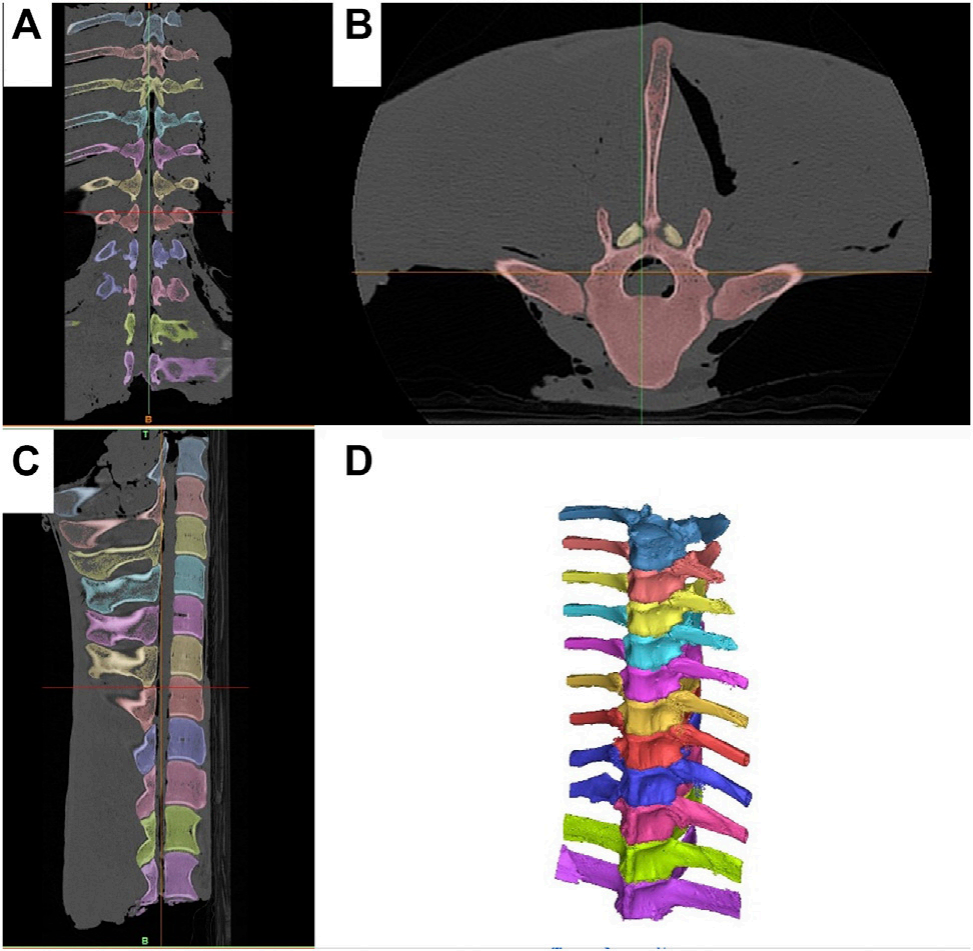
Segmentation of the T11-L1 spine segments. Each vertebra is segmented separately and denoted in a different color. (A) Dorsal CT scan view. (B) Axial CT scan view, (C) Sagittal CT scan view. (D) 3D reconstruction of each individual spinous process.
